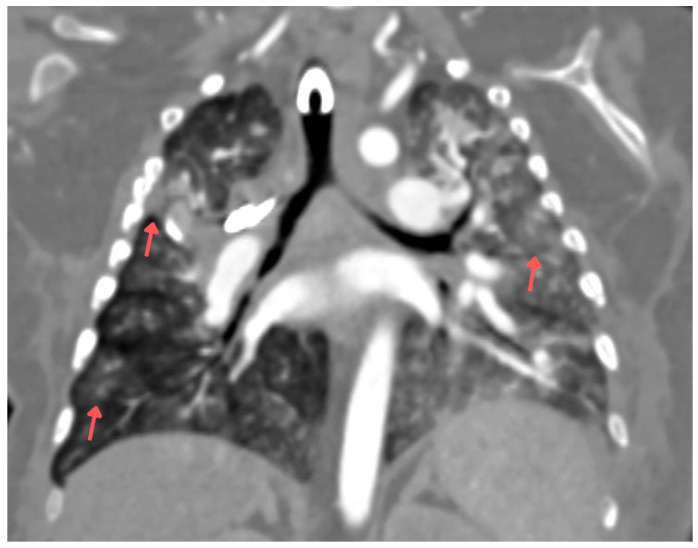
Contrast-enhanced chest CT (coronal section) performed 6 months after the initial examination (10 days prior to death). Some lesions were marked with arrows.
